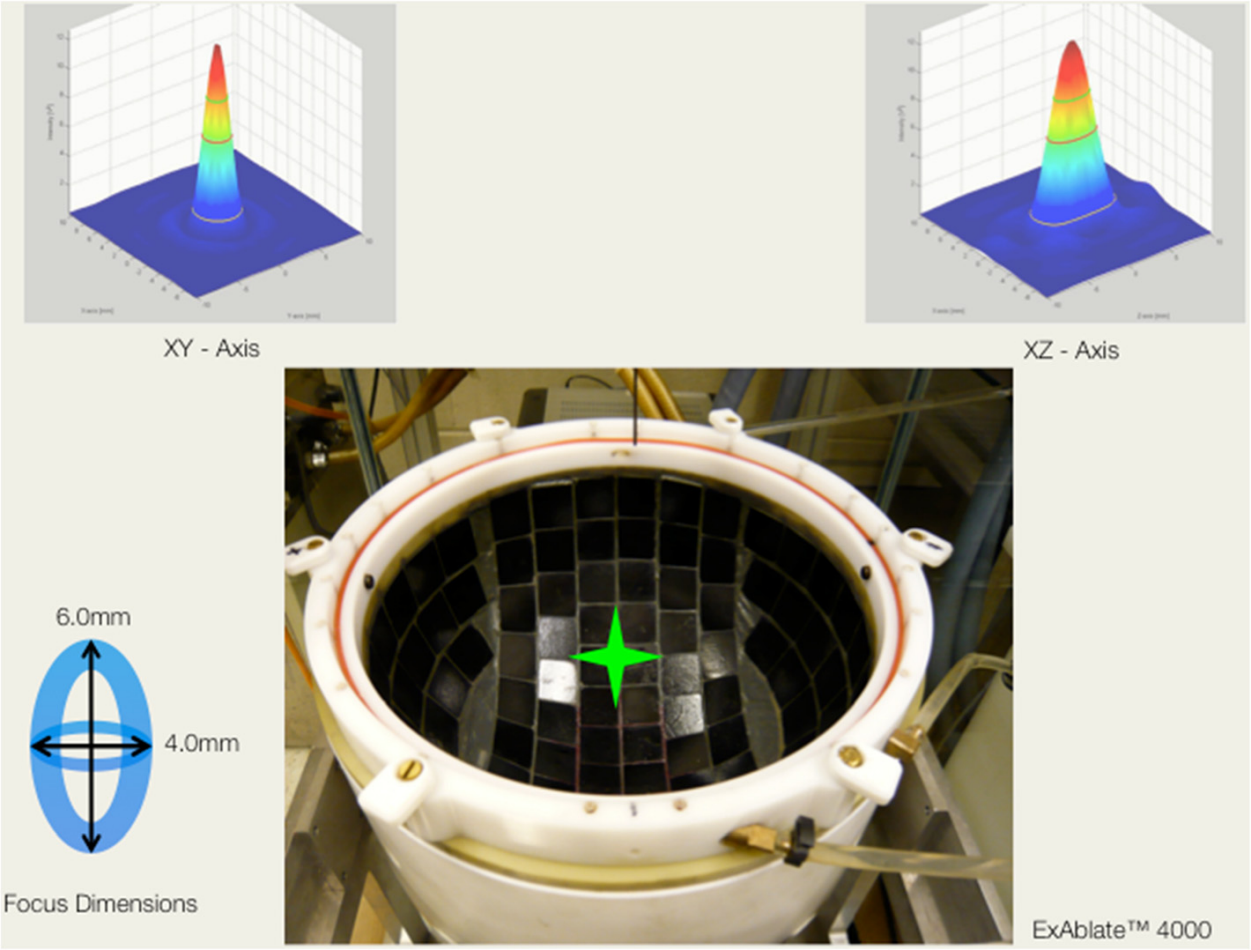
Top view inside the hemispheric transducer of the ExAblate™ 4000 HIFU headsystem. Each black tile contains nine single ultrasound-transmitting elements (total *N* = 1,000). All elements transmit towards the center of the transducer (green star), creating a sharp focus beam of 4.0-mm diameter in lateral and 6.0 mm in elevational orientation. Parametric images of the focus, based on acoustic measurements, are given in *XY* (upper left) and *Z* (upper right) orientations.
